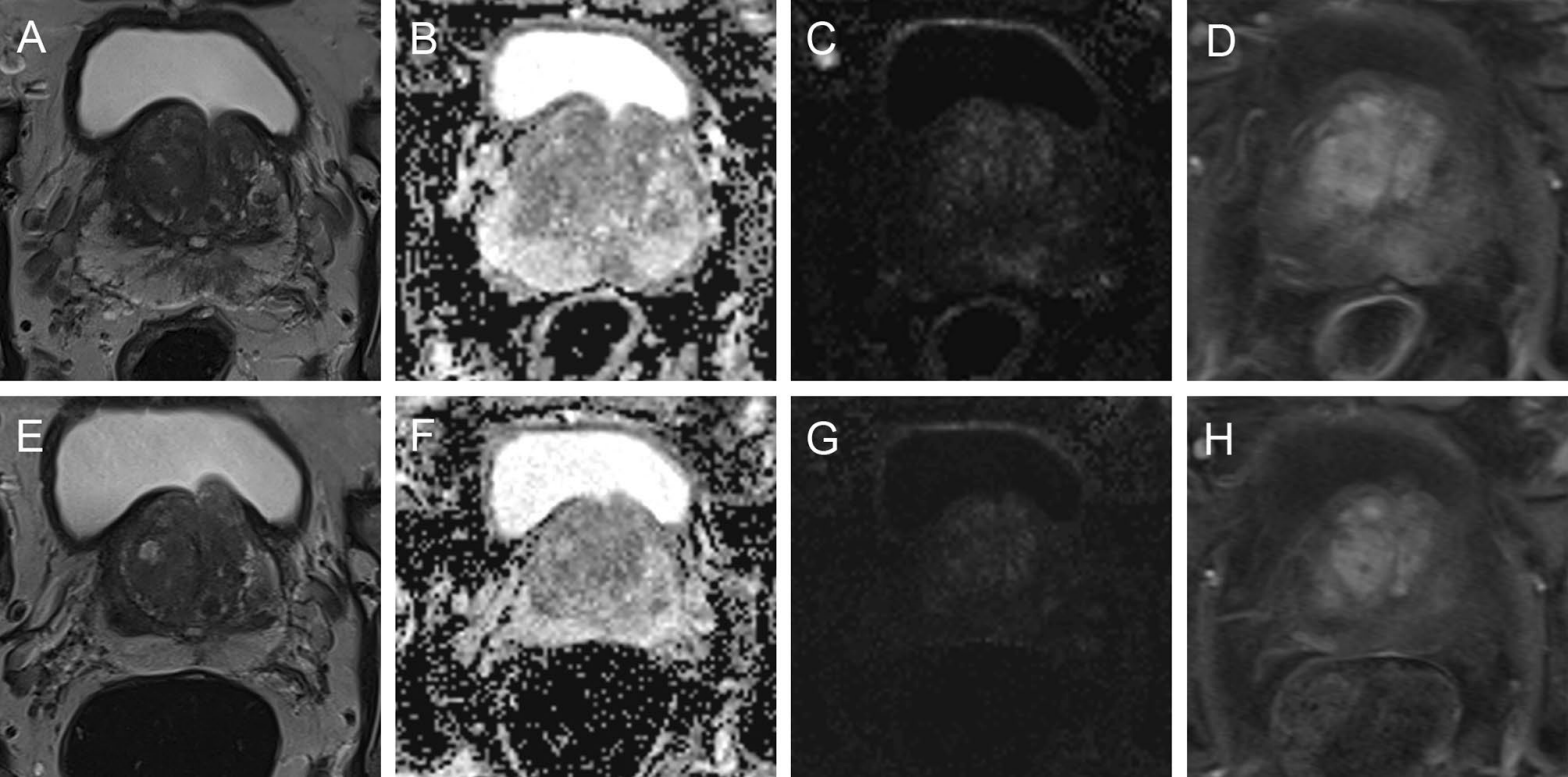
64-year-old patient with rising PSA 12.2 ng/m. Initial MRI examination: Axial T2-weighted image (A) showed a non-circumscribed, rounded, moderate hypointensity in the left lateral peripheral zone with focal discrete hypointense ADC signal (B), focal discrete hyperintense signal on high b-value DWI (C) and discrete correlating contrast enhancement in DCE (D), assessed as PI-RADS category 3. Follow-up MRI after 38 months: Axial T2-weighted image (E) showed only residual changes, no ADC reduction (F), no more hyperintense signal on high b-value DWI (G), and no contrast enhancement (H). The PSA decreased (6.6 ng/mL) and mpMRI was downgraded to PIRADS category 2.
